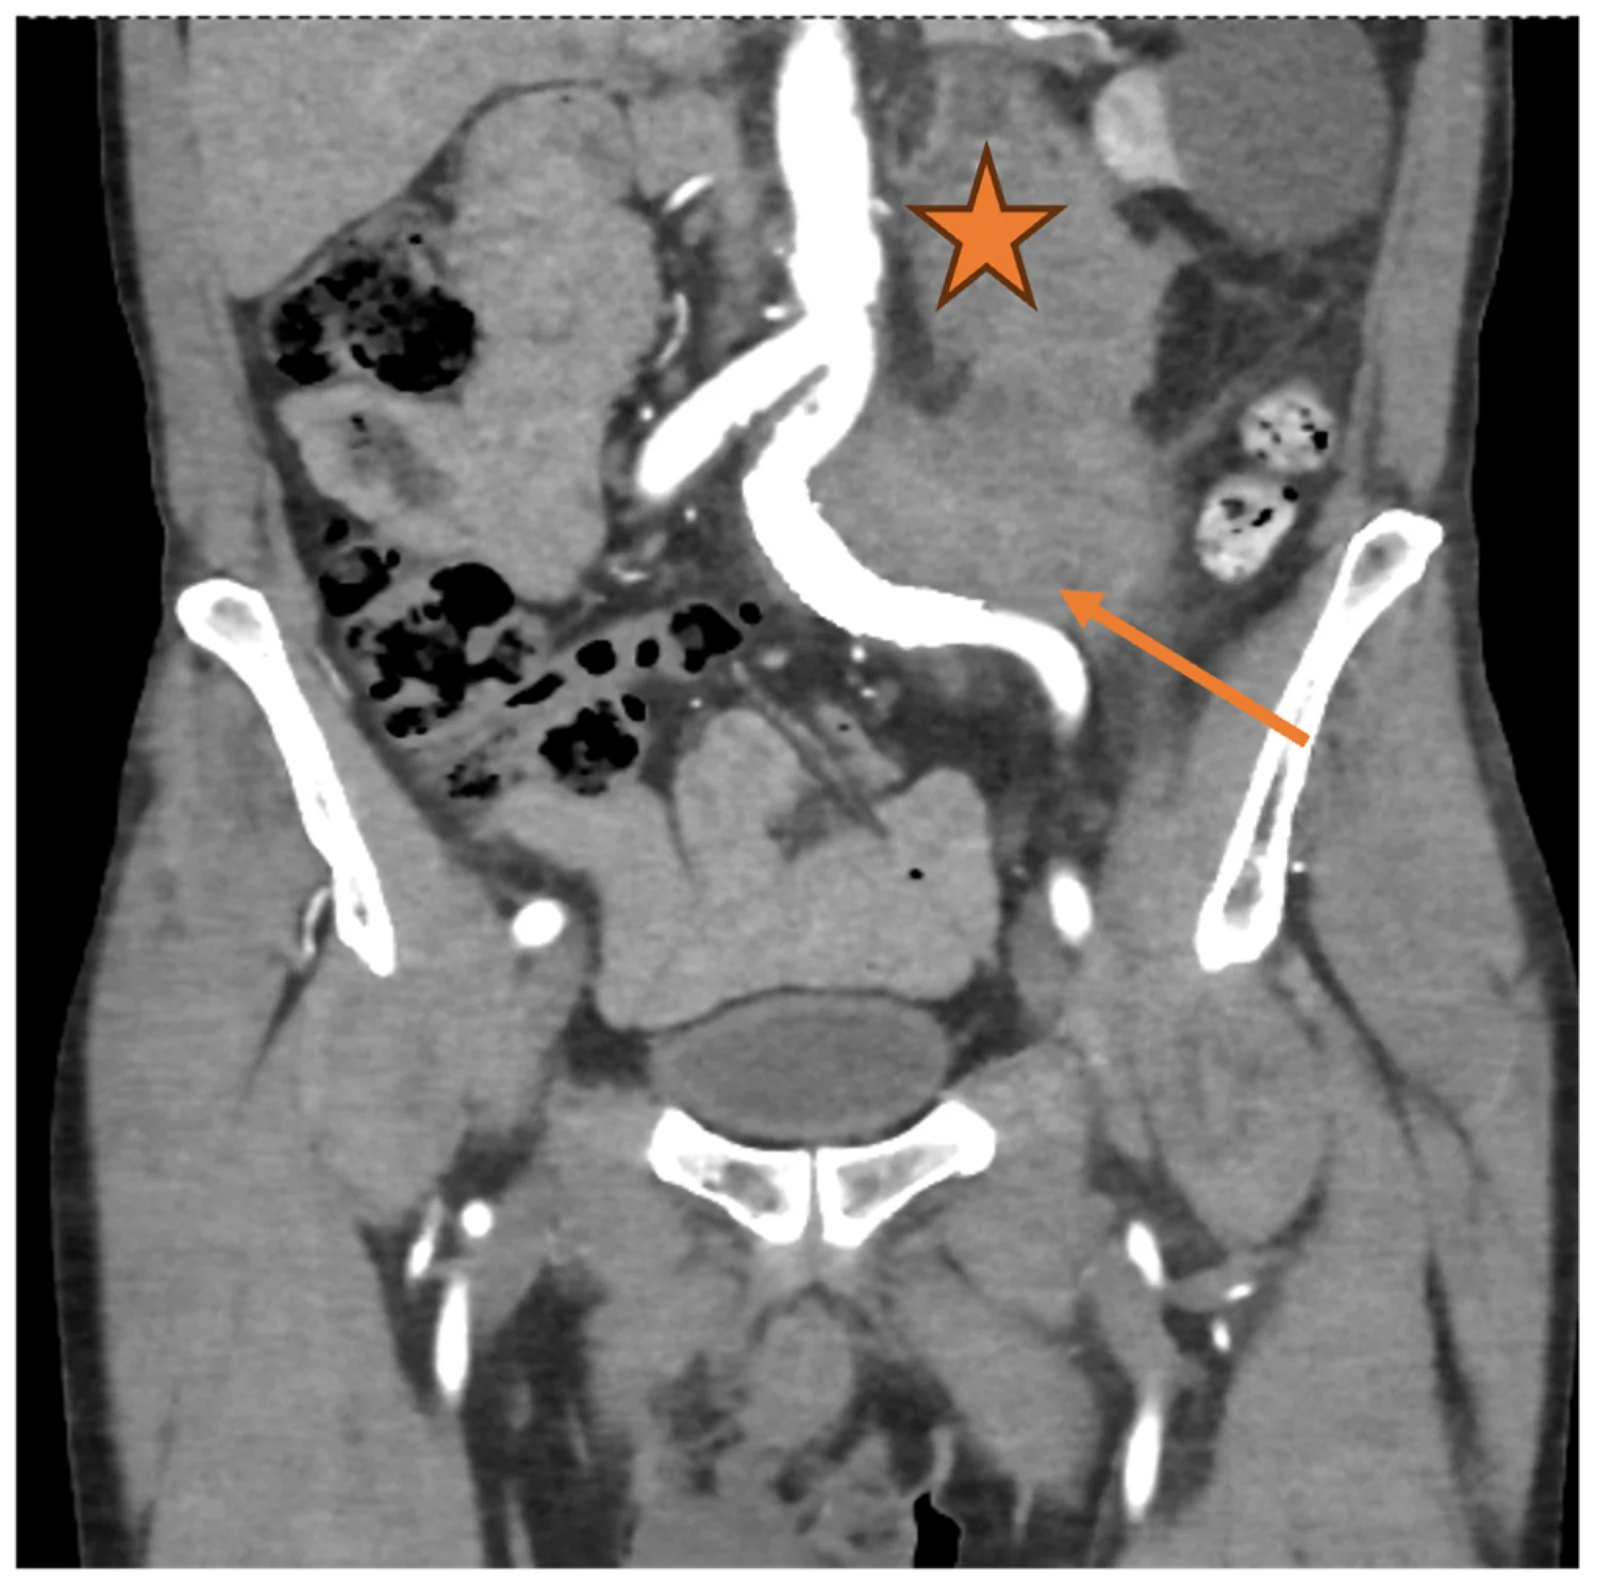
Post-revascularization angio-CT (coronal view) showing no extravasation of the contrast in the aneurysmal lumen (orange arrow) and a slight reduction in the size of the retroperitoneal hematoma (orange star).
